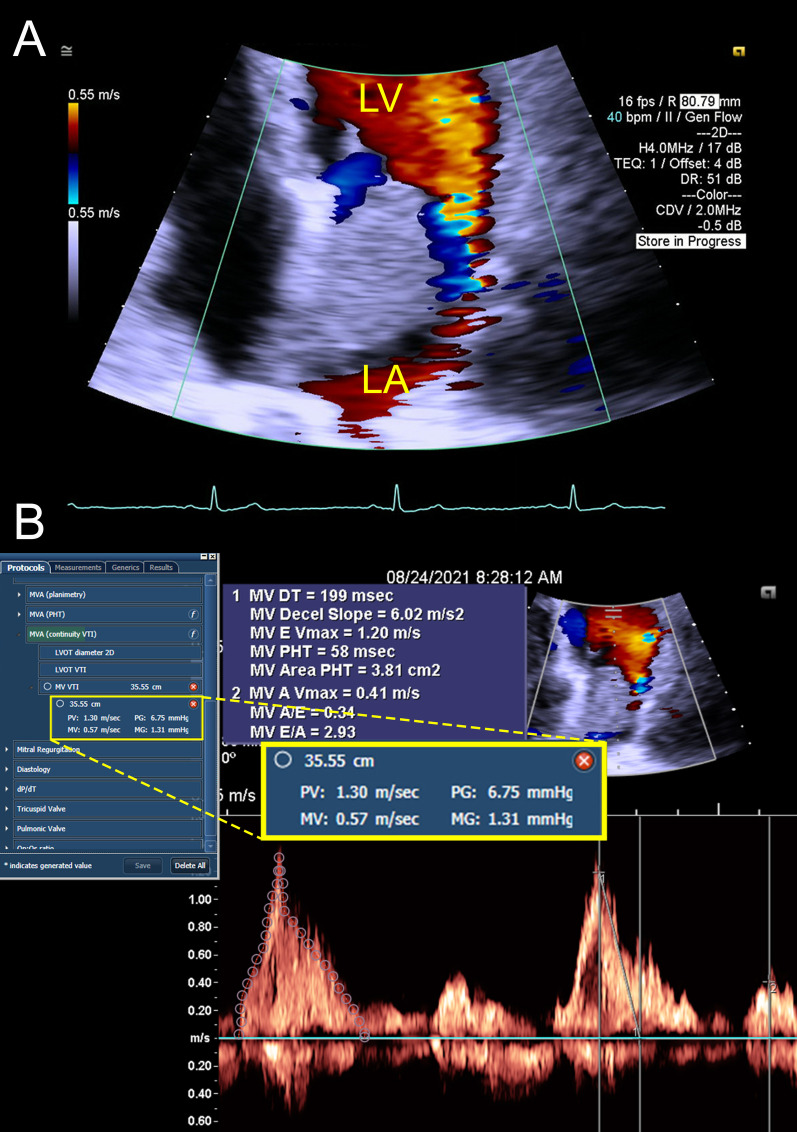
Doppler Flow and Calculated Mean Pressure Gradient Across Mitral Valve. a Doppler Image of Myxoma showing flow around the myxoma. b Mean pressure gradient across mitral valve measured in apical four chamber with Velocity Time Integral method, revealing Mean Pressure Gradient of 1.31 mmHg
